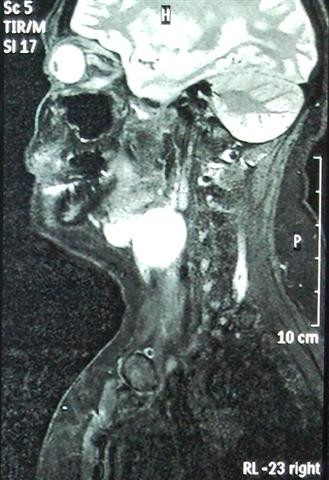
Axial T2-weighted MRI image showing a large lesion partially obstructing the supraglottic airway structures.
